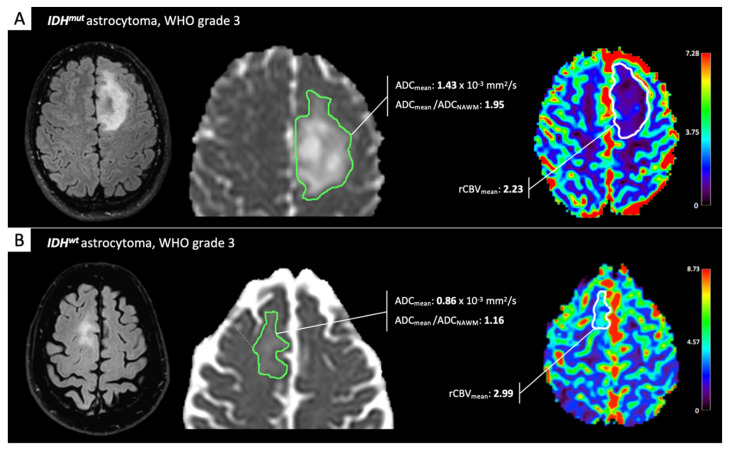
Perfusion and diffusion assessment of lower-grade glioma (LGG). MRI datasets were retrieved from the archive of our Institution. Diffusion MRI (dMRI) and perfusion-weighted imaging (PWI) features from one isocitrate dehydrogenase mutant (IDH^mut^)-LGG (A) and one IDH wild type (IDH^wt^)-LGG (B) were evaluated through a clinically feasible single-slice region of interest (ROI) approach. IDH^wt^-LGG exhibits more “aggressive” aMRI features, in particular a mean apparent diffusion coefficient (ADC_mean_)/normal-appearing white matter (ADC_NAWM_) ratio lower than 1.8, the proposed cutoff for IDH^wt^ status prediction by Maynard et al. [27] and Thust et al. [28].
